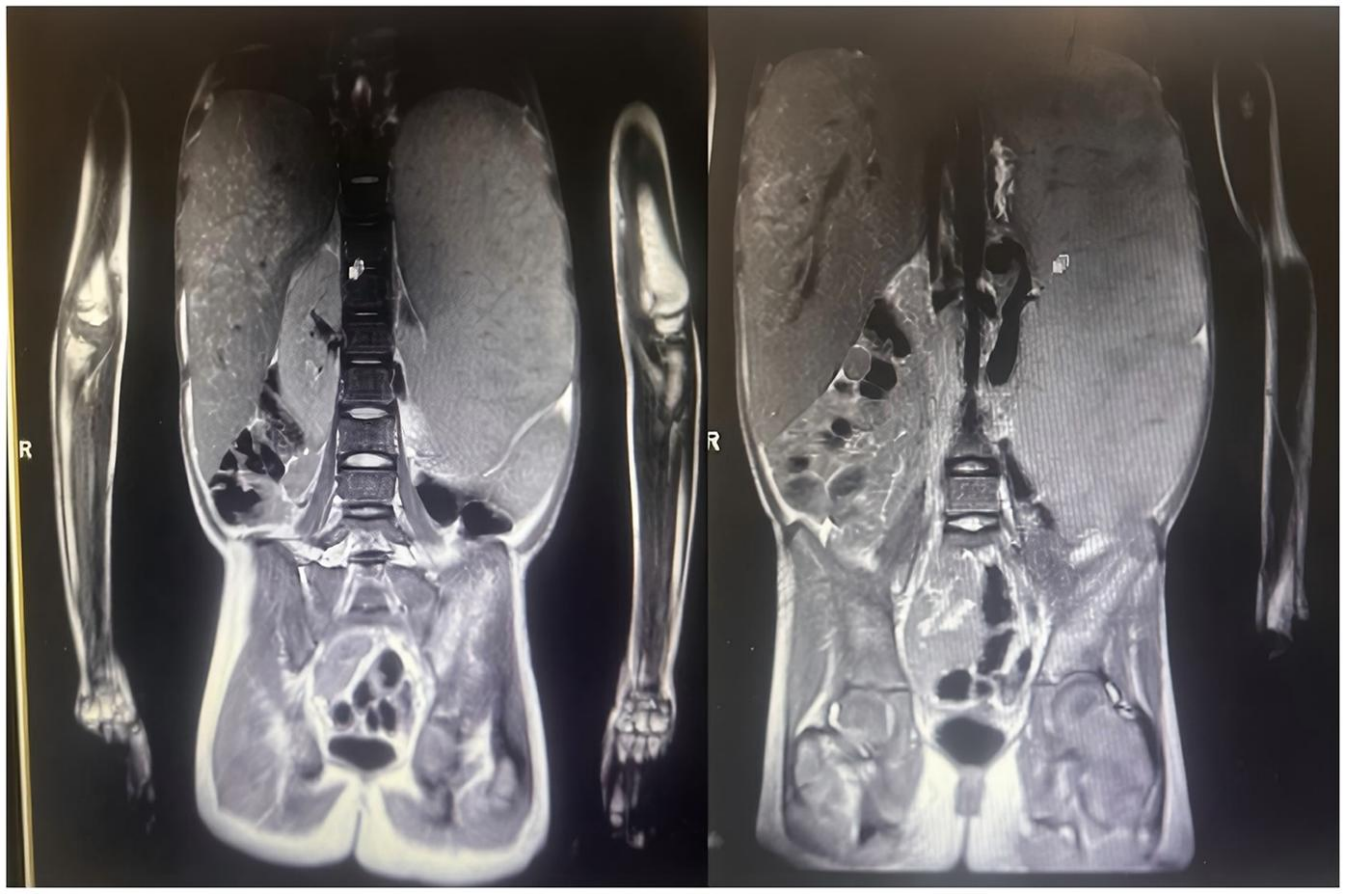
Abdominal MRI (2024-01-19) Liver: enlarged liver volume, with no significant widening of the liver fissures. No apparent dilation of the intrahepatic bile ducts. The liver's right lobe shows heterogeneous signals, with patchy areas exhibiting slightly high signals on T2-weighted imaging (T2WI). No significant abnormalities detected in the bile ducts. Spleen: Enlarged spleen, with homogeneous signals in the parenchyma. The splenic hilum shows dilation of the veins, with the widest part measuring approximately 12 mm. Kidneys: Both kidneys exhibit normal morphology and signals, with no significant abnormalities. Left Hip Bone: High signal on T2WI, indicating possible pathology such as edema or inflammation.
